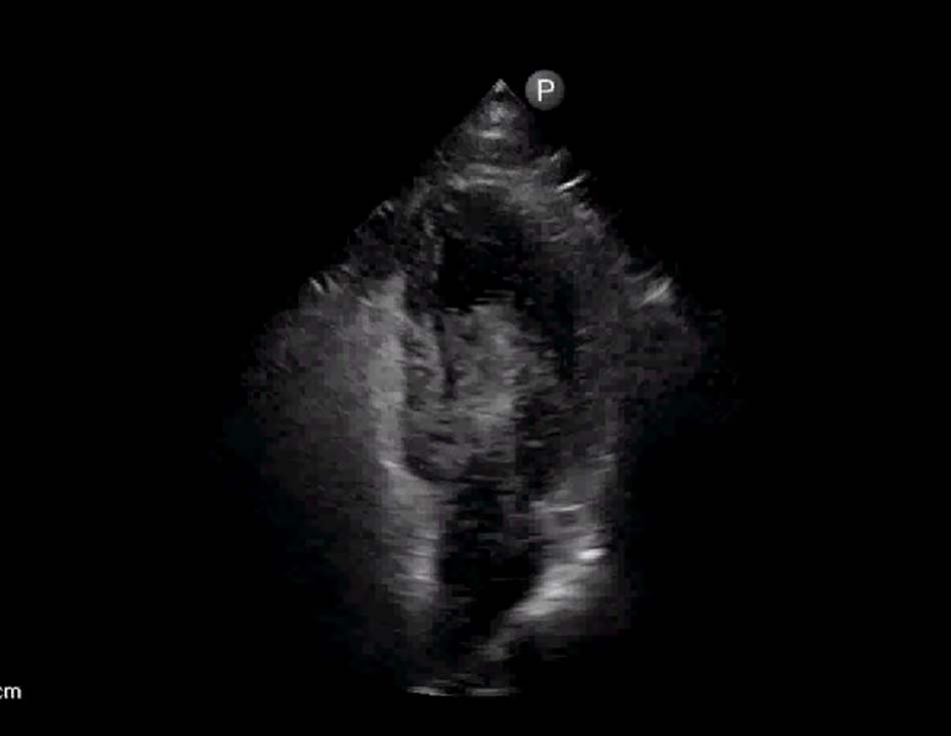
Apical four chamber view with early right to left shunt by agitated saline study.
